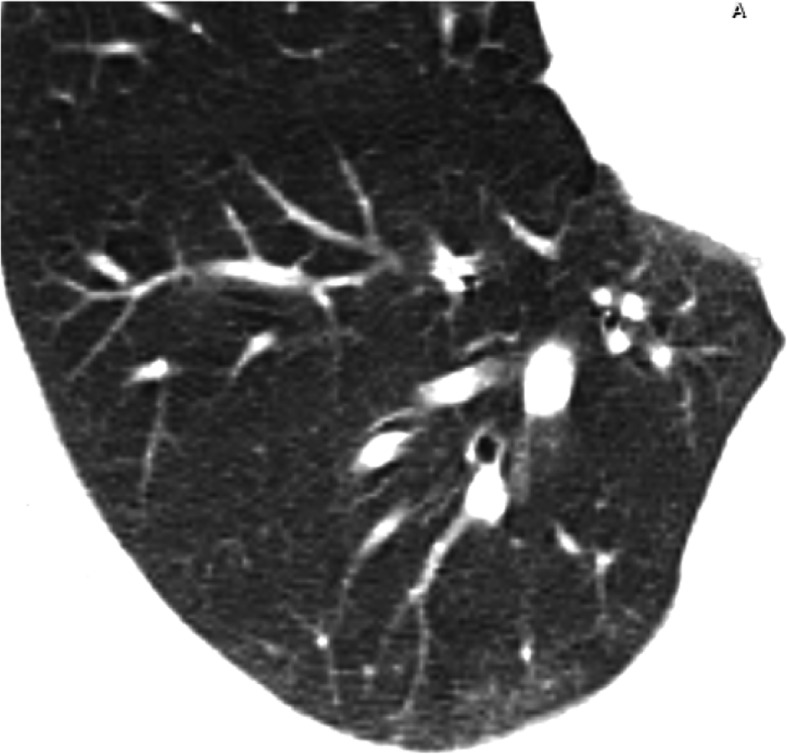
Male patient 38 years old with COVID-19. Magnified axial CT image shows right lower lobe subpleural ground glass opacification with prominent vessels within
